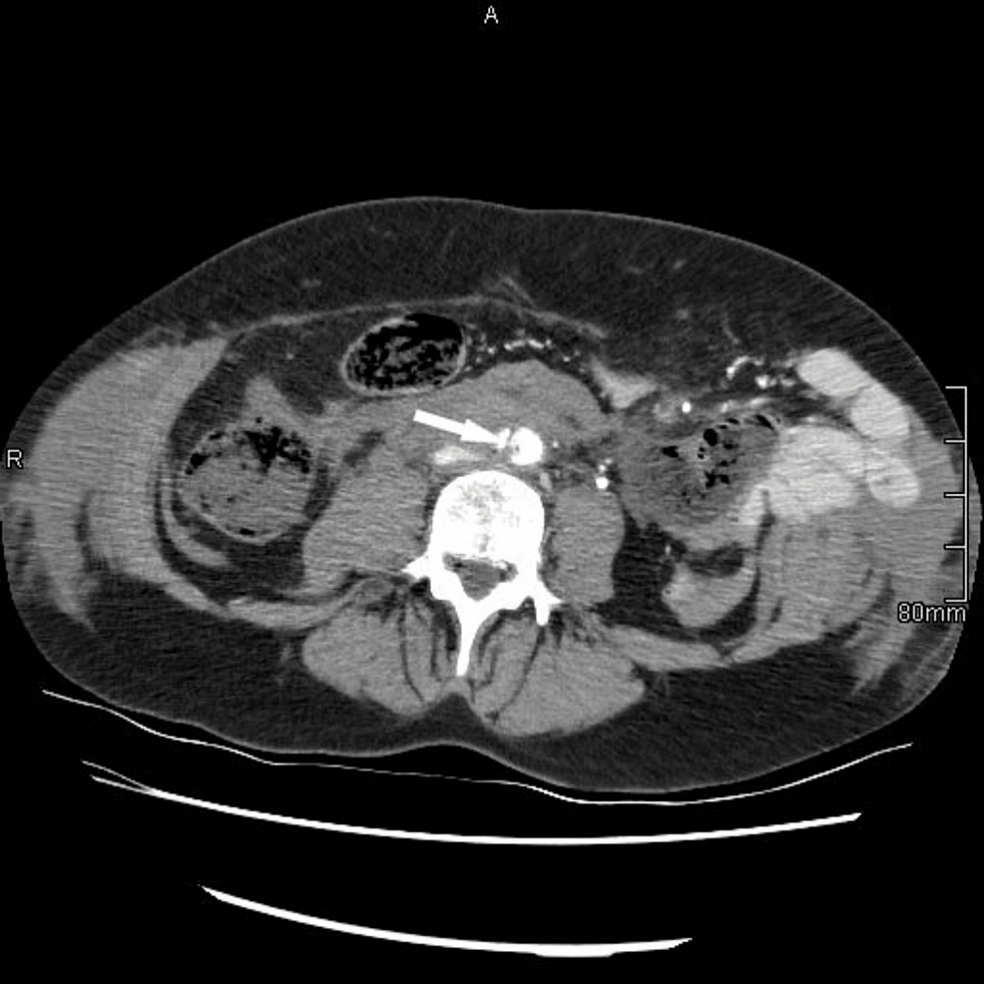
Initial CT, axial image, images show rupture of the anterior abdominal wall with mesenteric injury and dissection (arrow) of the distal part of the abdominal aorta. Periaortic changes are not hematoma but, as confirmed during surgery, displaced bowel loops
